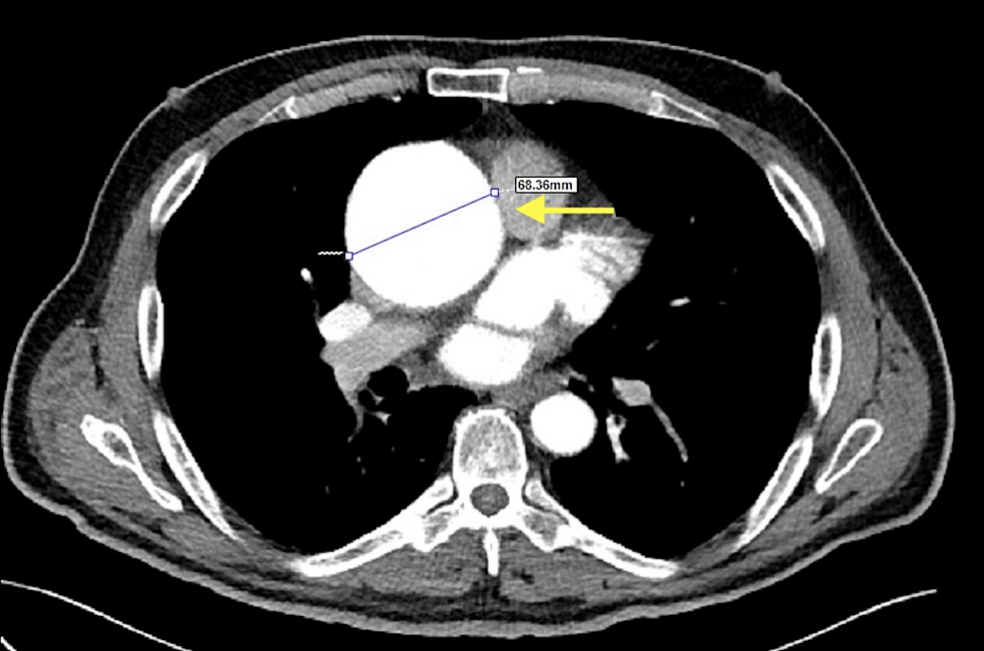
Ascending aortic aneurysm and transverse diameter of 69 mm on CT angiography
